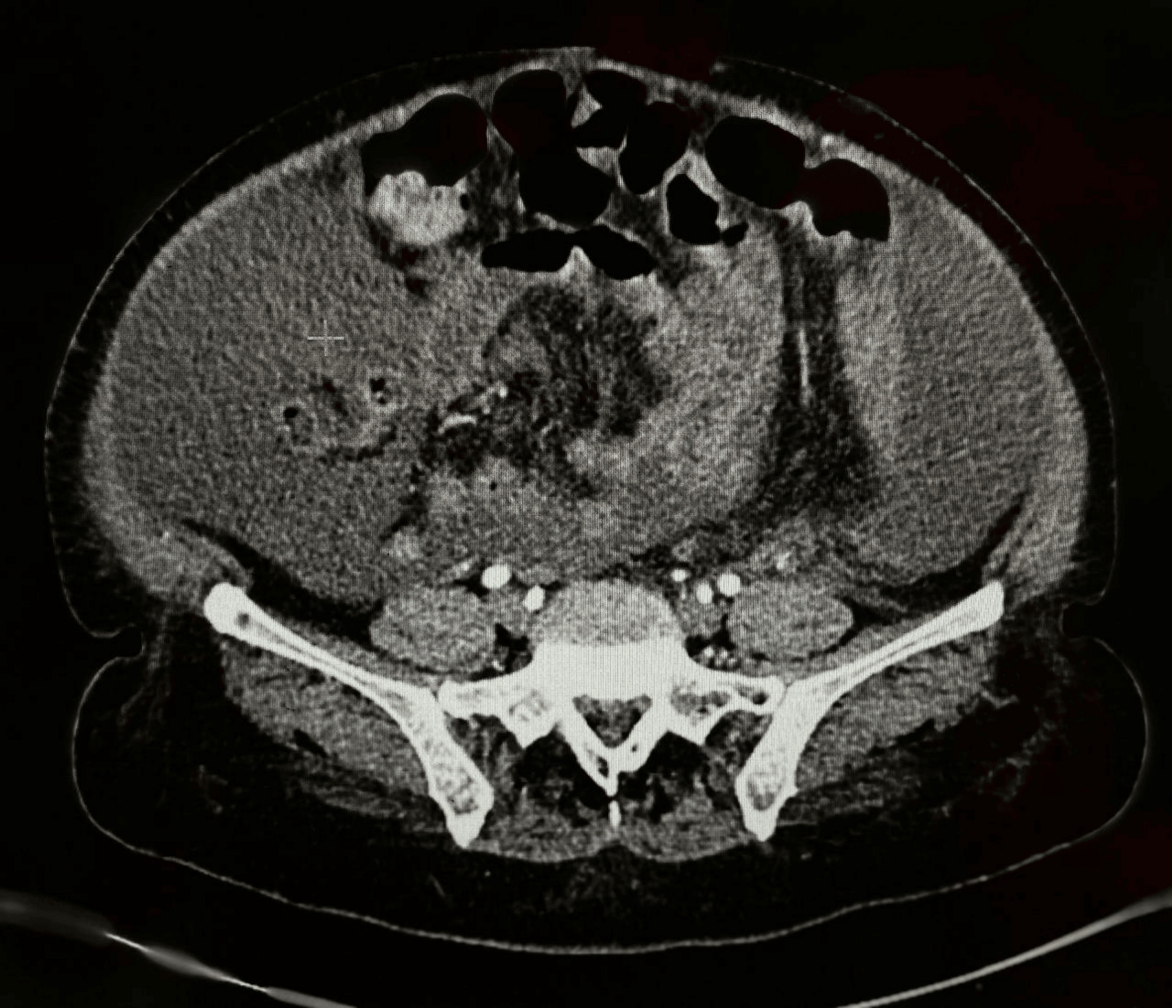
CT scan of the abdomen and pelvis revealing massive ascites without evidence of cancerous lesions
